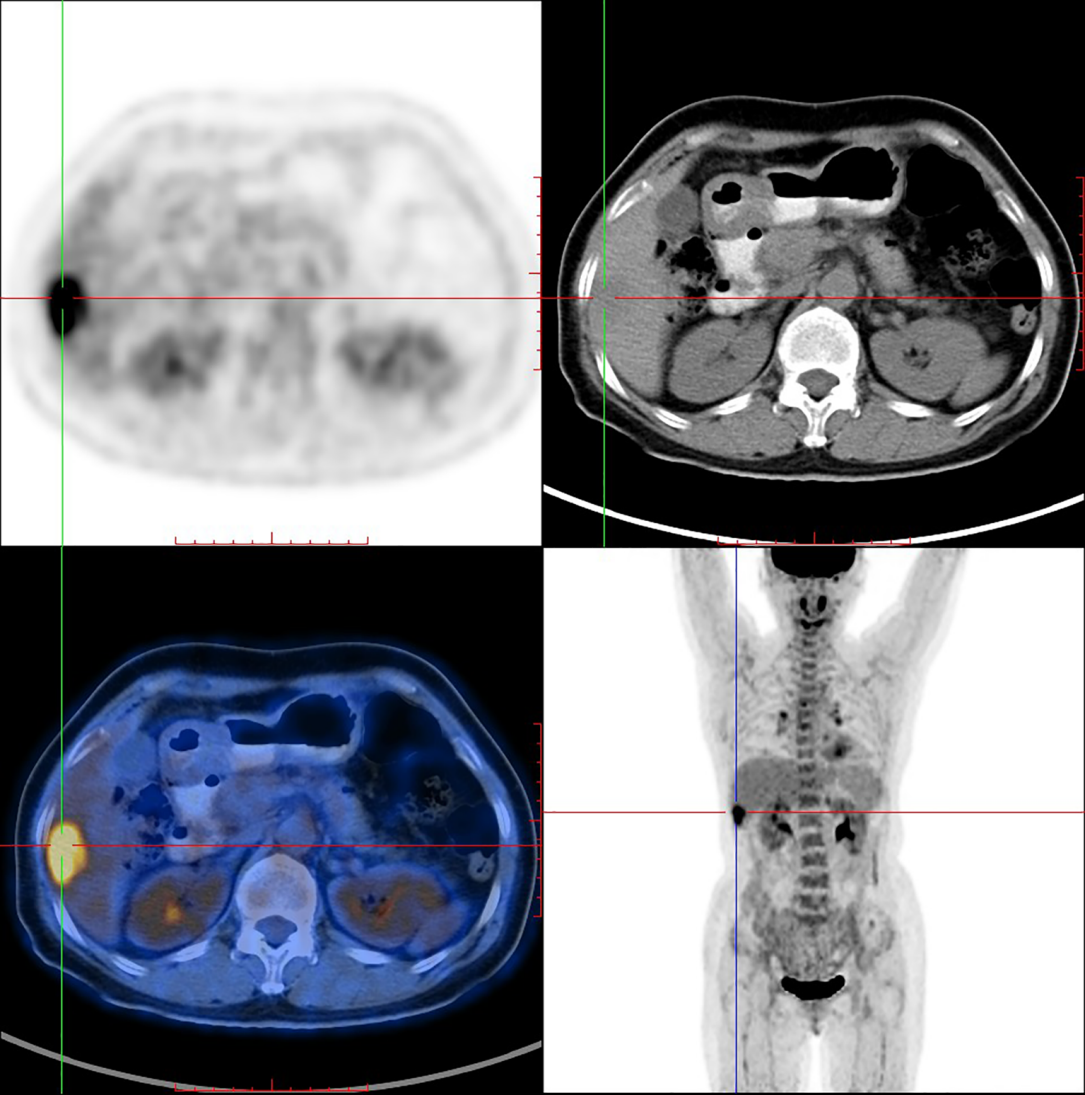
^18^F-FDG PET/CT imaging of the same female IDC patient (54 y).
